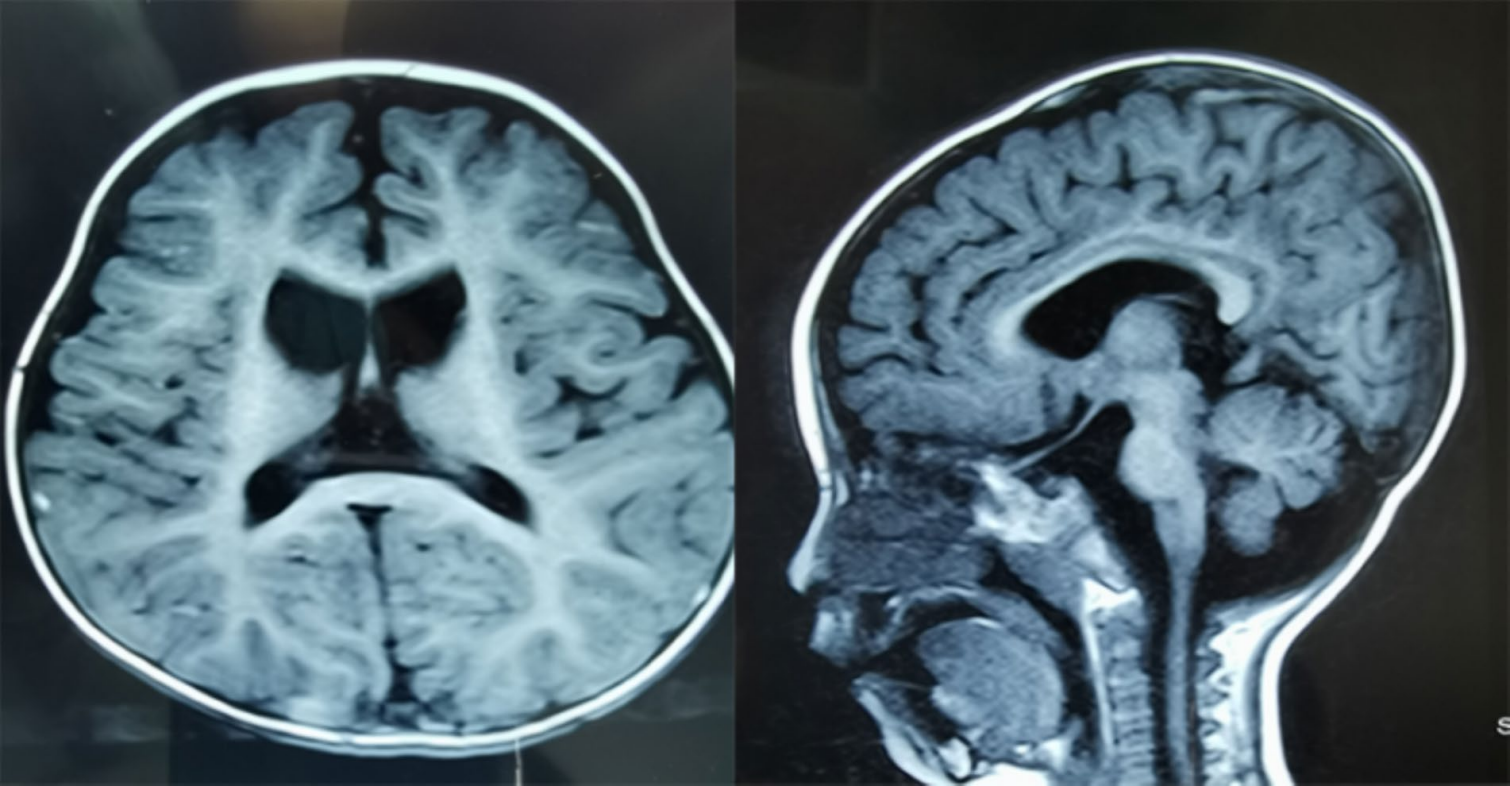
Brain MRI of the PI exhibiting bilateral hyperintensity within the putamina nuclei and caudate (left panel), as well as mild medullary and cerebellar atrophy (right panel).
